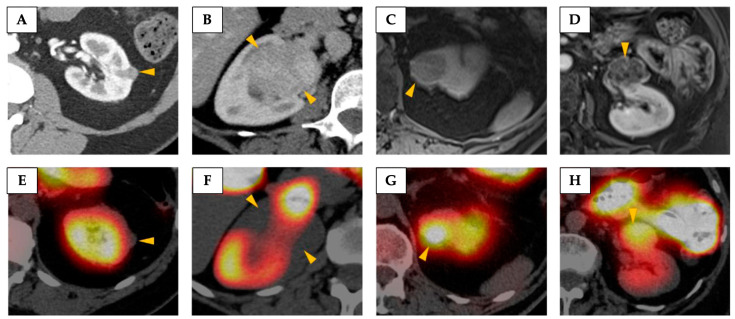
A demonstration of the high variability of ^99m^Tc-sestamibi uptake in chromophobe renal cell carcinomas (chRCCs). Axial images from contrast-enhanced CT (A,B) and post-contrast T1-weighted fat-saturated MRI (C,D) showing representative images of the four chRCCs included in the study performed by Rowe et al. [99]. (E–H) Corresponding ^99m^Tc-sestamibi SPECT/CT images of the same tumours demonstrating the highly variable uptake seen in this tumour type. Tumours are denoted by yellow arrowheads. The tumours in (A/E) and (B/F) were qualitatively considered cold, whereas the tumours in (C/G) and (D/H) were qualitatively considered hot. Reprinted, under a CC BY 4.0 license, from reference [99].
